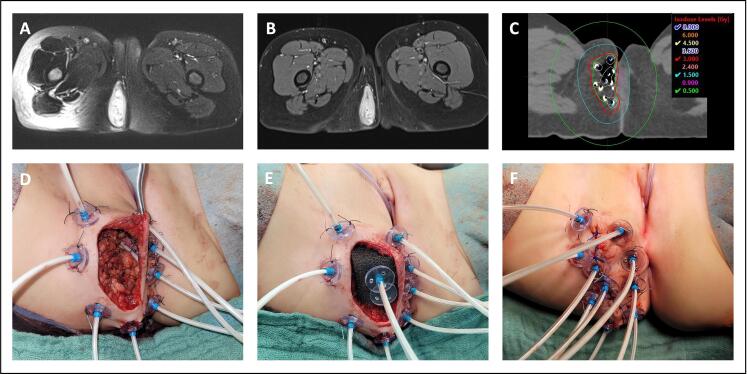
Organ-preserving resection combined with brachytherapy in a 50-month-old girl (patient #6) with embryonal, perianal rhabdomyosarcoma. A) Initial MRI. B) Preoperative MRI. C) Radiation treatment planning showing target volume (inner green line), organs at risk (vagina (purple line) and rectum (brown line)) and isodose lines per fraction. D) Situs after tumor resection and placement of brachytherapy tubes. E) Situs after additional placement of a sponge with brachytherapy tubes into the wound cavity. F) Position of the tubes after closure of the skin over the sponge.
